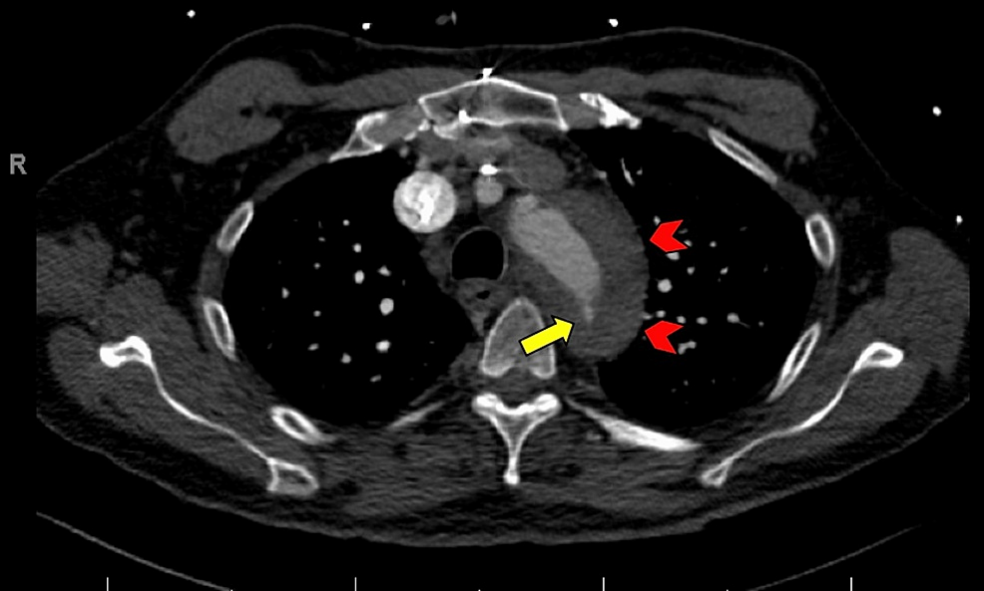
CT (axial view) demonstrating the aortic tear (yellow arrow) and surrounding hematoma formation (red arrowheads).
